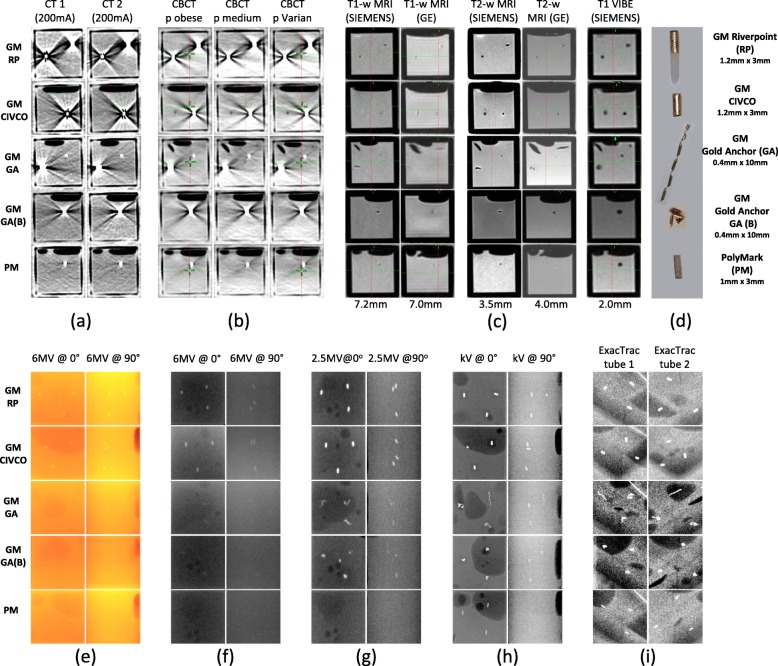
Cross-sectional/planar images of boxes with different FM acquired using different imaging modalities. a–b Volumetric X-ray scans, c MRI scans, and e–i Planar X-ray images. d Different fiducial markers used in this study, diameter x length
